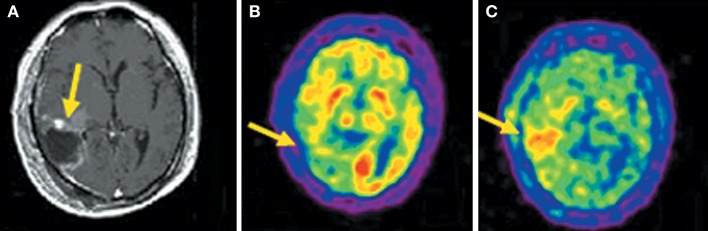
Images (Axial) of a 45-year-old man with a history of attempted resection of World Health Organization (WHO) grade glioma. (A) Subsequent new abnormal enhancing lesion (arrow) on Magnetic resonance (MRI). (B)
